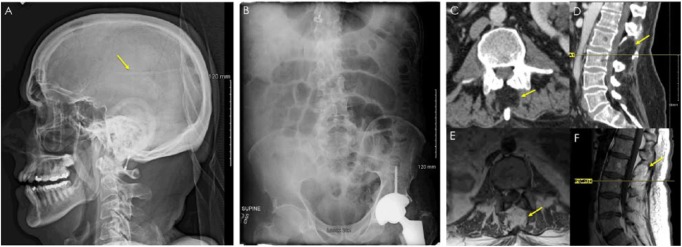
Initial imaging investigations. Proximal remnant of a ventricular atrial shunt is visible on lateral head view of X-ray shuntogram (A). No radiopaque abdominal shunt can be found on abdominal X-ray (B), although the left hip arthroplasty is visible. Computed tomography with intravenous contrast reveals an unknown lumbar process (C, D). Magnetic resonance imaging without contrast enhancement reveals the lumbar defect has the same T1 weighted signal as adipose tissue (E, F), suggesting possible postoperative changes of unknown chronicity.
